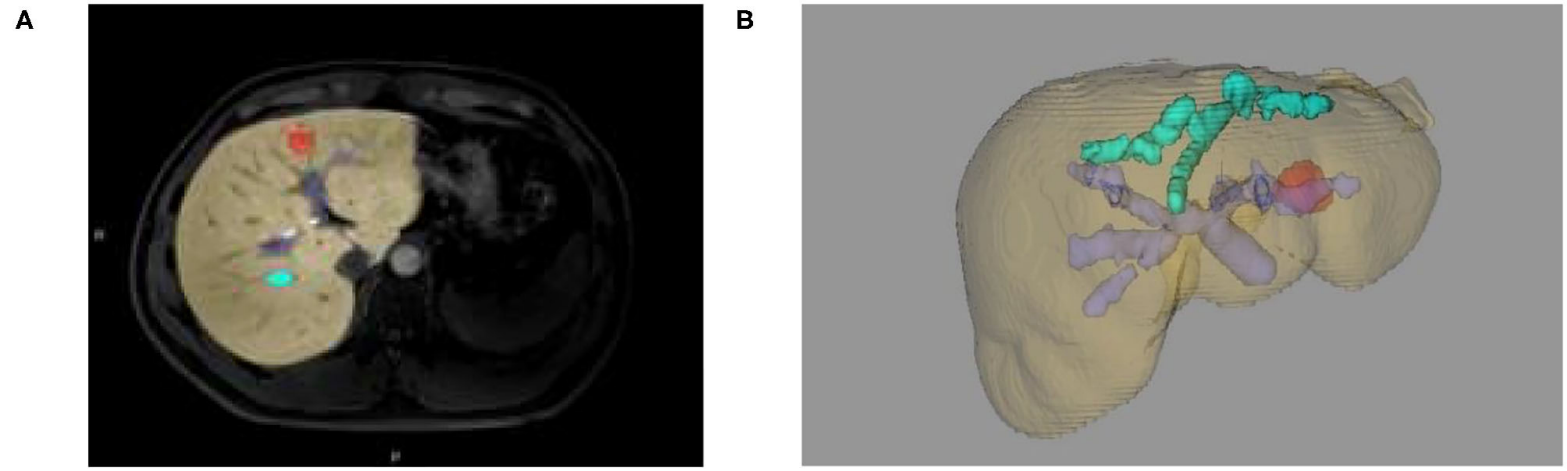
(A) Two-dimensional MRI enhanced image, (B) 3D reconstruction of liver and tumor based on growth model on MRI data.
